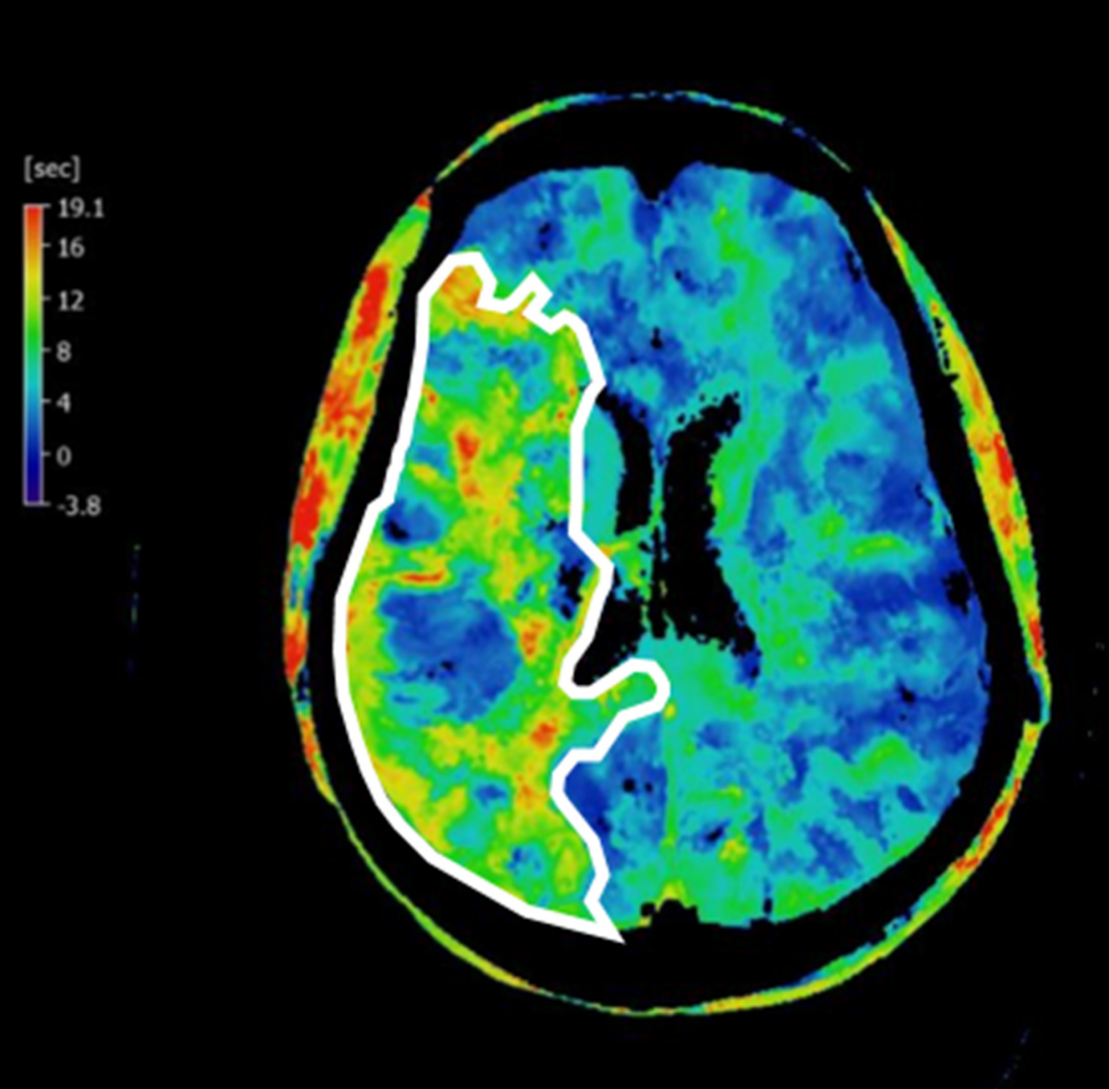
Time to Peak Computed Tomography Perfusion Study A time to peak computed tomography study demonstrates delayed blood flow in the penumbra, within the right middle cerebral artery distribution (white outline).
